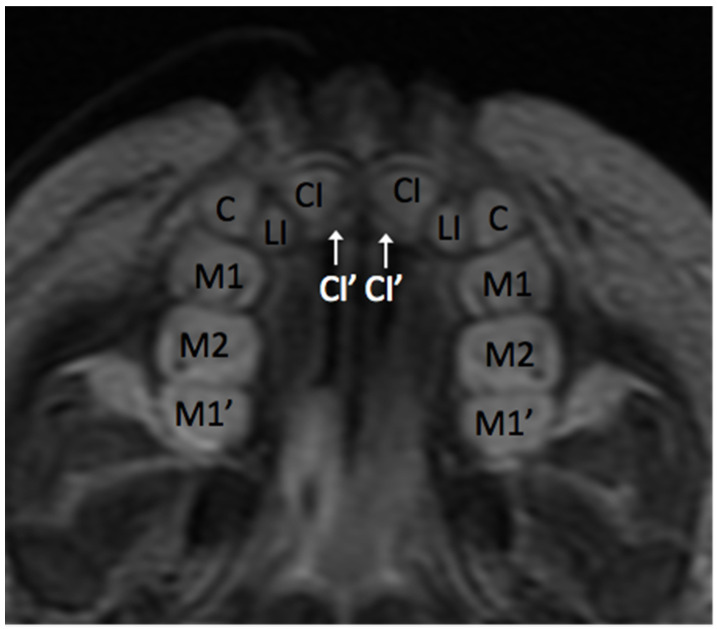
Axial section T2WI MRI: primary maxillary teeth in maturation stage with permanent maxillary central incisors (CI′) and first molars (M1′) in pre-appositional stages.
